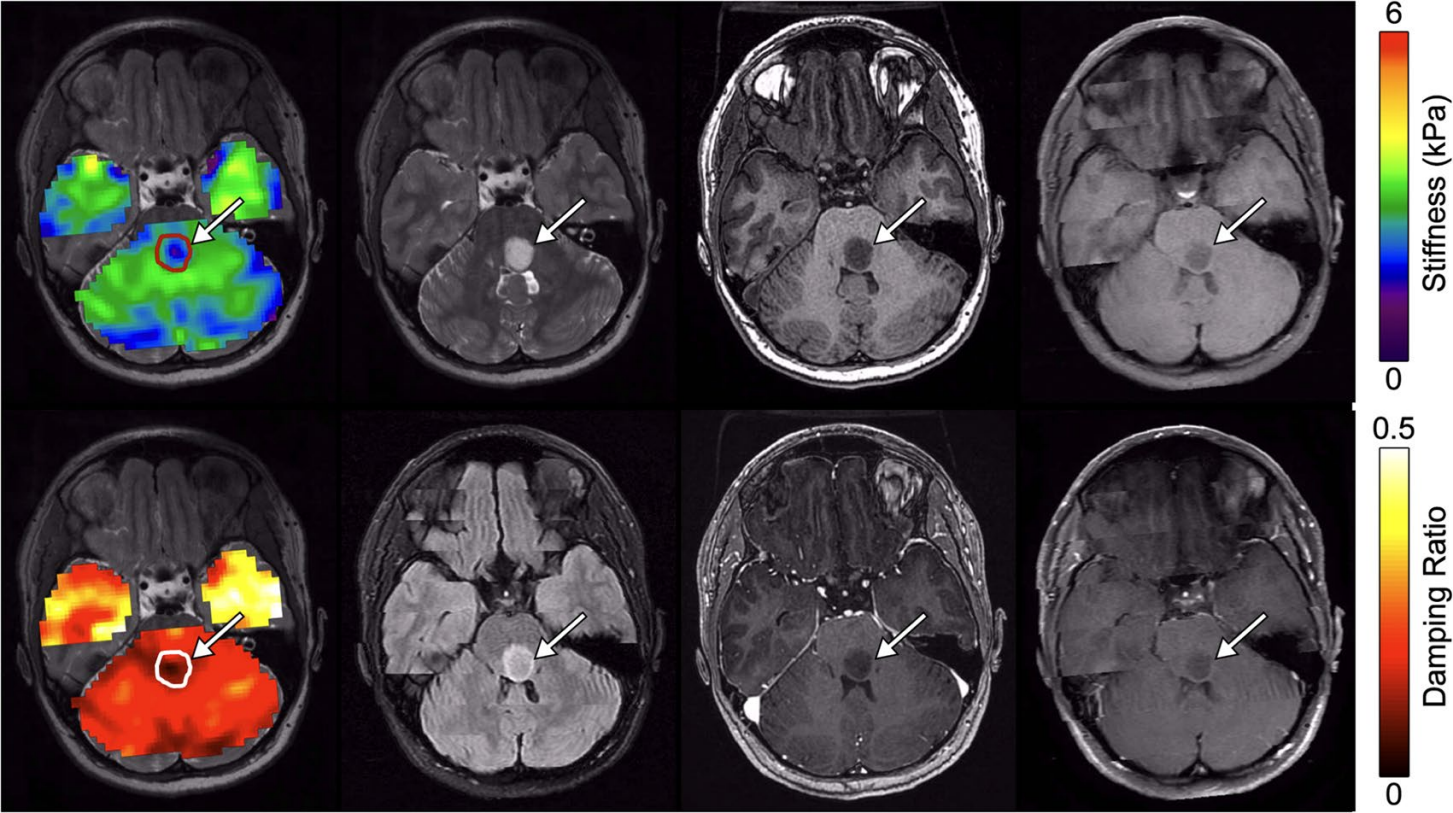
16-year-old female with a pontine glioma and NF1 (Patient 6). Top row, left-to-right: Stiffness map, T_2_-CUBE, T_1_-BRAVO, MEMP; Bottom row, left-to-right: Damping ratio map, T_2_-FLAIR, post contrast T_1_-BRAVO, post contrast MEMP
